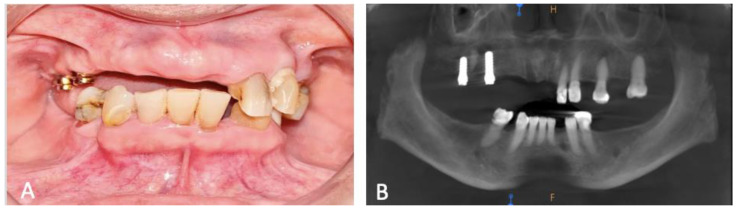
Pre-operative assessment (A) Clinical appearance of anterior maxilla at baseline. (B) Orthopantomagram (OPG). H stands for height and F stands for floor indication superior and inferior border of image.
